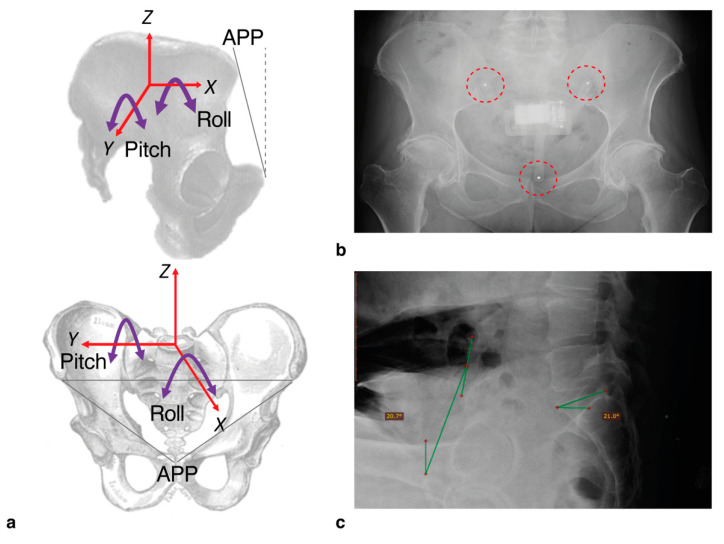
With the IMU device in place, we were able to measure pelvic tilt according to the axes shown (a). For this study, we only measured pitch (i.e., tilt in the anterior-posterior direction, around the *Y*-axis). For the radiograph assessments, the tilt was measured according to the angle of the anterior pelvic plane (APP) defined by the plane between the anterior superior iliac spines and the pubic tubercles, relative to the vertical (dashed line). To check positioning of the device following capture, 3 mm steel bearings were inserted into the legs which appeared clearly on radiographs ((b); highlighted by dashed circles). An example radiograph showing the annotated measure of tilt based on APP relative to vertical (green lines) is shown in (c). Sacral slope was also calculated but not used in this study.
